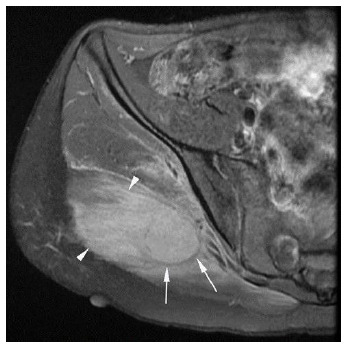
Axial MRI pelvis – significant surrounding oedema (arrowheads), extending laterally to the greater trochanter and medially into the sciatic notch. A rim of hypointense calcifications (arrows) seen at the periphery of the lesion. Axial measurement is 3.4 x 2.5cm.
